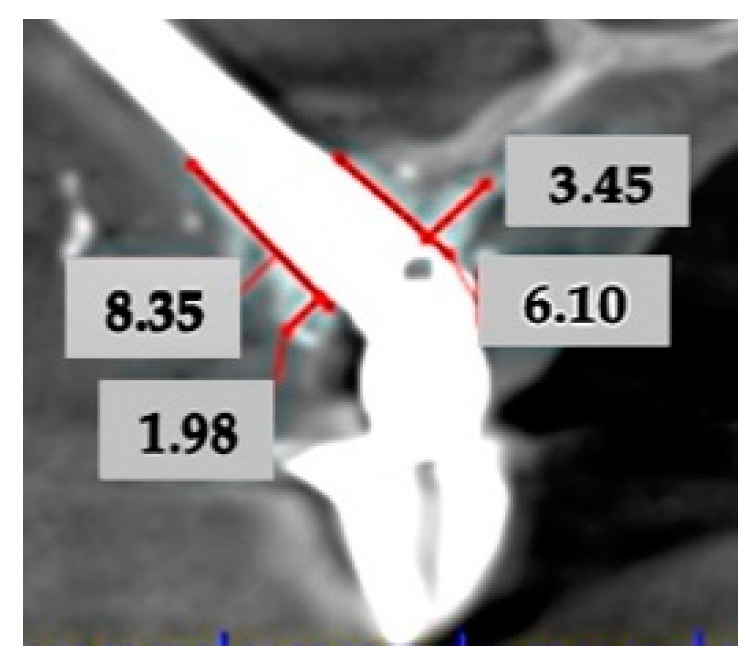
Bone gain measurement by CBCT at 6 months in two directions: through a perpendicular buccal and palatal lines to the implant axis (width) and through a parallel buccal and palatal lines to the implant axis at level of coronal part of the implant (length).
